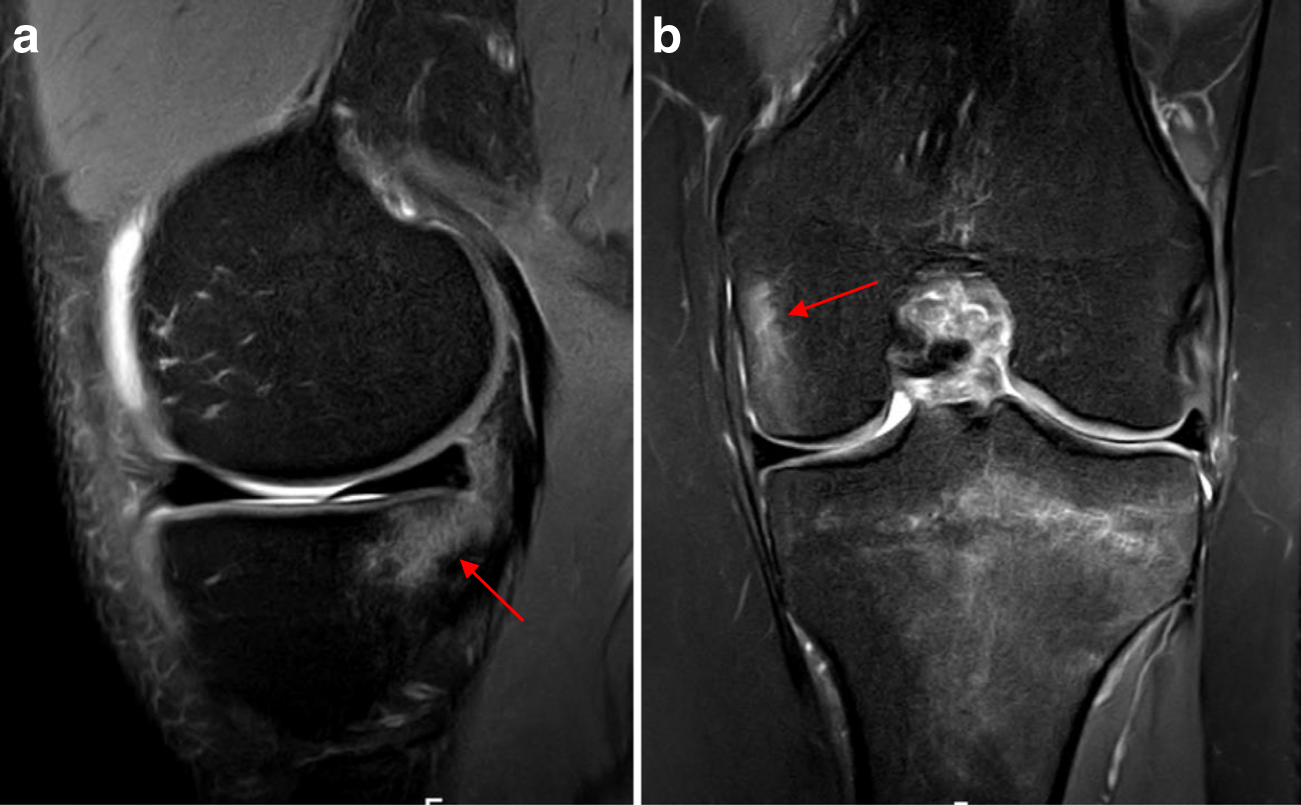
Posteromedial tibial (a) and medial femoral condyle (b) bone bruising (arrows) on sagittal and coronal proton density fat saturation magnetic resonance imaging sequence (left knee)
